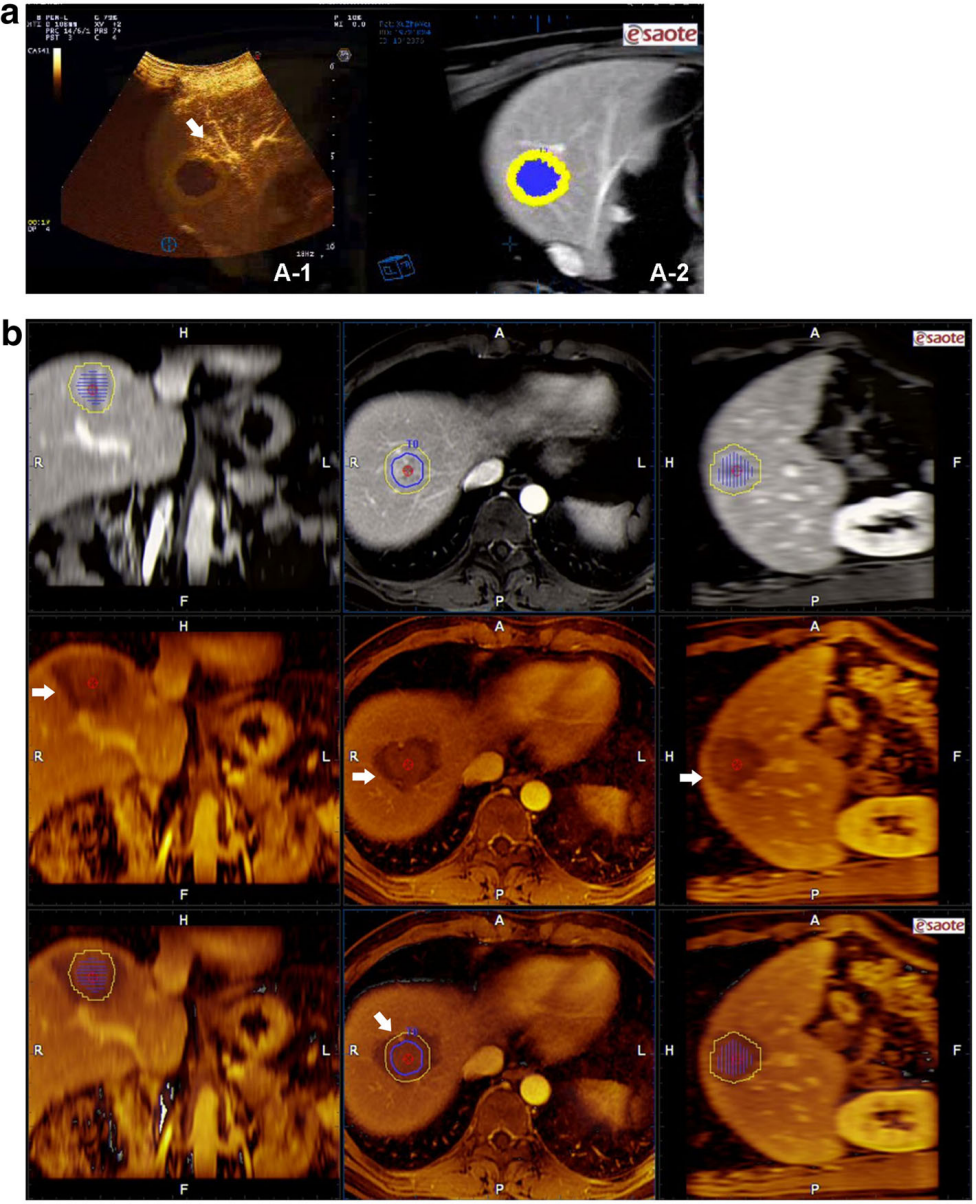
Medical images of case 2 in the clinical study. (A-2) Preoperative MR image (tumor shown in blue and 5-mm AM in yellow). (A-1) CEUS-MR fusion image showing that the area of ablation encompassed the tumor but not the entire AM due to the influence of vessels (*white arrow*). b MR-MR fusion image from the same patient. *Upper panel*: preoperative MR images (tumor shown in blue and 5-mm AM in yellow). *Middle panel*: postoperative MR images (arrow, area of ablation shown in dark color). *Lower panel*: MR-MR fusion images showing that the tumor and AM were not fully encompassed by the area of ablation (white arrow)
